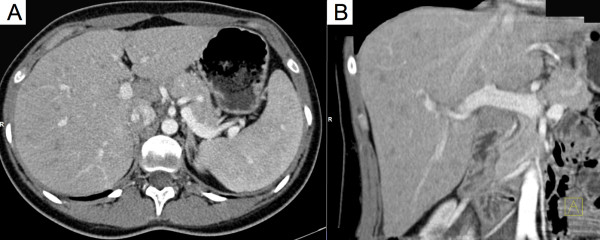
Radiographic findings of abdominal computed tomography during Katayama syndrome (axial (A) and coronal (B) reconstruction).
